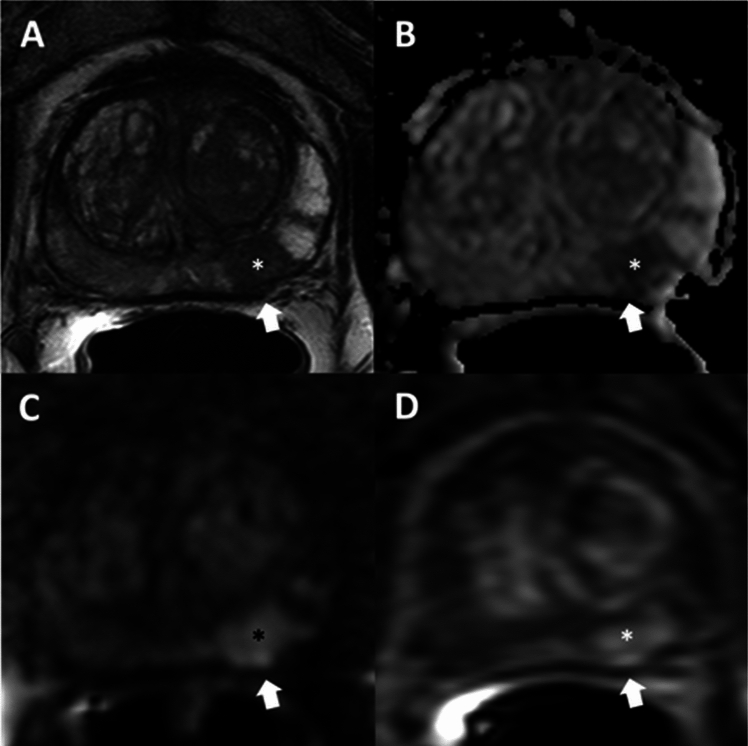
Multiparametric MRI of a 59-year-old patient with a serum prostate-specific antigen level of 6.1 ng/mL. AI classified T2WI as high-quality. The lesion (asterisk) was 1.5 cm in the left mid-base peripheral zone and assigned PI-RADS category 5. Slight capsular bulge was noted (NCI EPE grade = 1) (arrows). Radical prostatectomy showed Gleason score 9 (4 + 5) prostate adenocarcinoma with EPE, representing a true positive EPE call. Axial T2WI (A), apparent diffusion coefficient map (B), high b-value (b = 1500 s/mm^2^) diffusion weighted imaging (C), dynamic contrast enhanced imaging (D). AI artificial intelligence, T2WI T2-weighted imaging, PI-RADS Prostate Imaging Reporting and Data System, NCI National Cancer Institute, EPE extraprostatic extension
